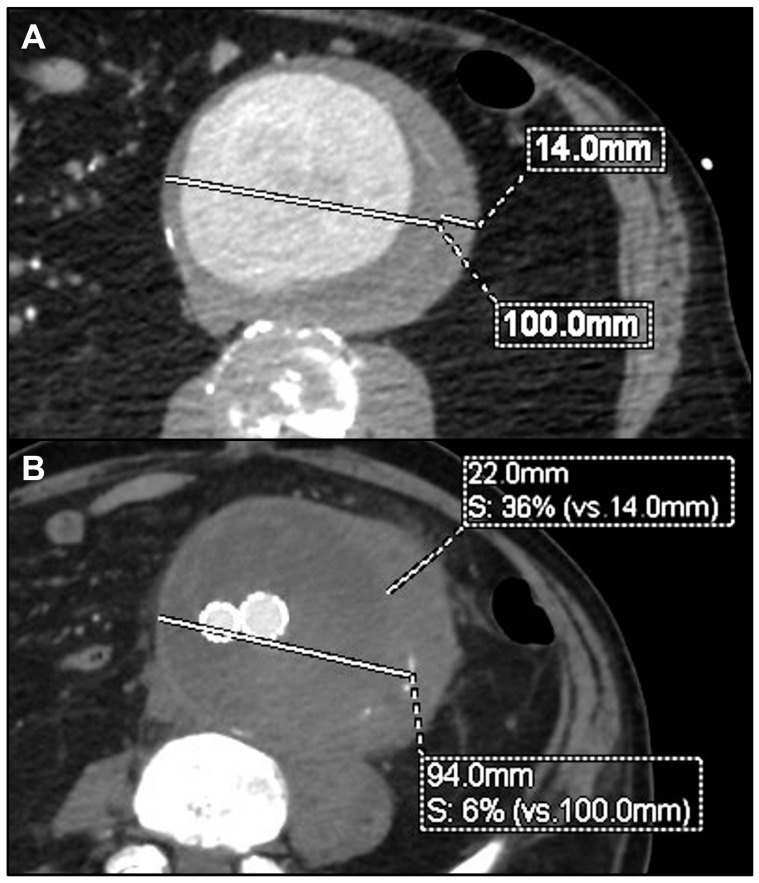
Preoperative computed tomography angiography (CTA) of symptomatic rapidly growing 100 mm infrarenal aneurysm (A) and 3-month postoperative CTA showing aneurysm’s shrinkage but significant growth of the peri-aortic inflammatory tissue (B).
